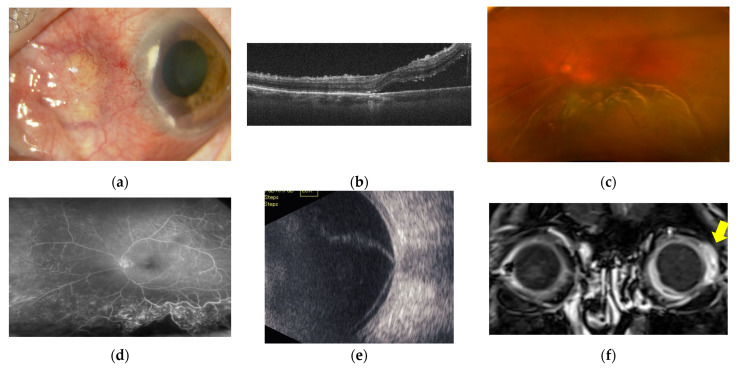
Case 3: Clinical appearance of anterior necrotizing scleritis, OCT, Colour fundus imaging, FFA, B-mode ultrasonography and MRI in the right eye. (a) Photograph of the anterior segment of the left eye at the first visit, showing necrotising scleritis and corneal marginal infiltration. (b) OCT shows the neuroepithelial layer as raised and wrinkled, with underlying fluid. (c) Colour fundus imaging shows papilloedema and extensive SRD including the fovea. (d) FFA shows retinal vasculitis consistent with SRD. (e) B-mode ultrasonography reveals increased scleral thickening (T-signatures) and extensive SRD. (f) Contrast-enhanced MRI shows contrast enhancement localised to the ocular wall of the left eye (yellow arrows).
